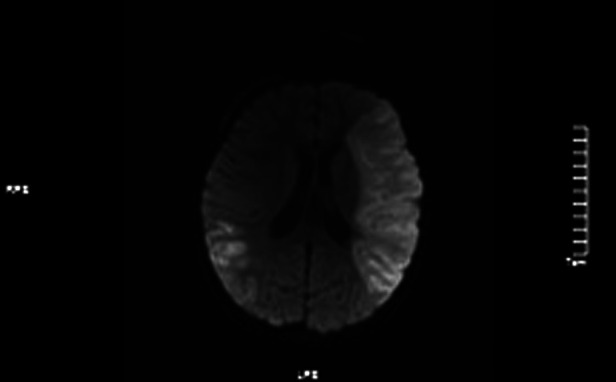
DWI images showed high signals in the left frontotem poral lobe and the right temporal.
